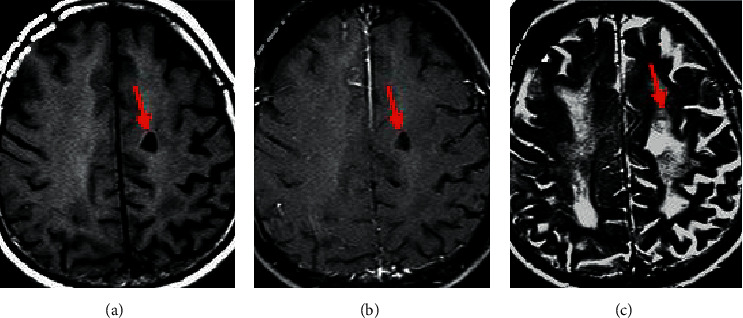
MRI image of asymptomatic cerebral infarction of a male patient aged 45 years. (a), (b), (c) The T1-weighted image, T2-weighted image, and flair manifestation, respectively.
